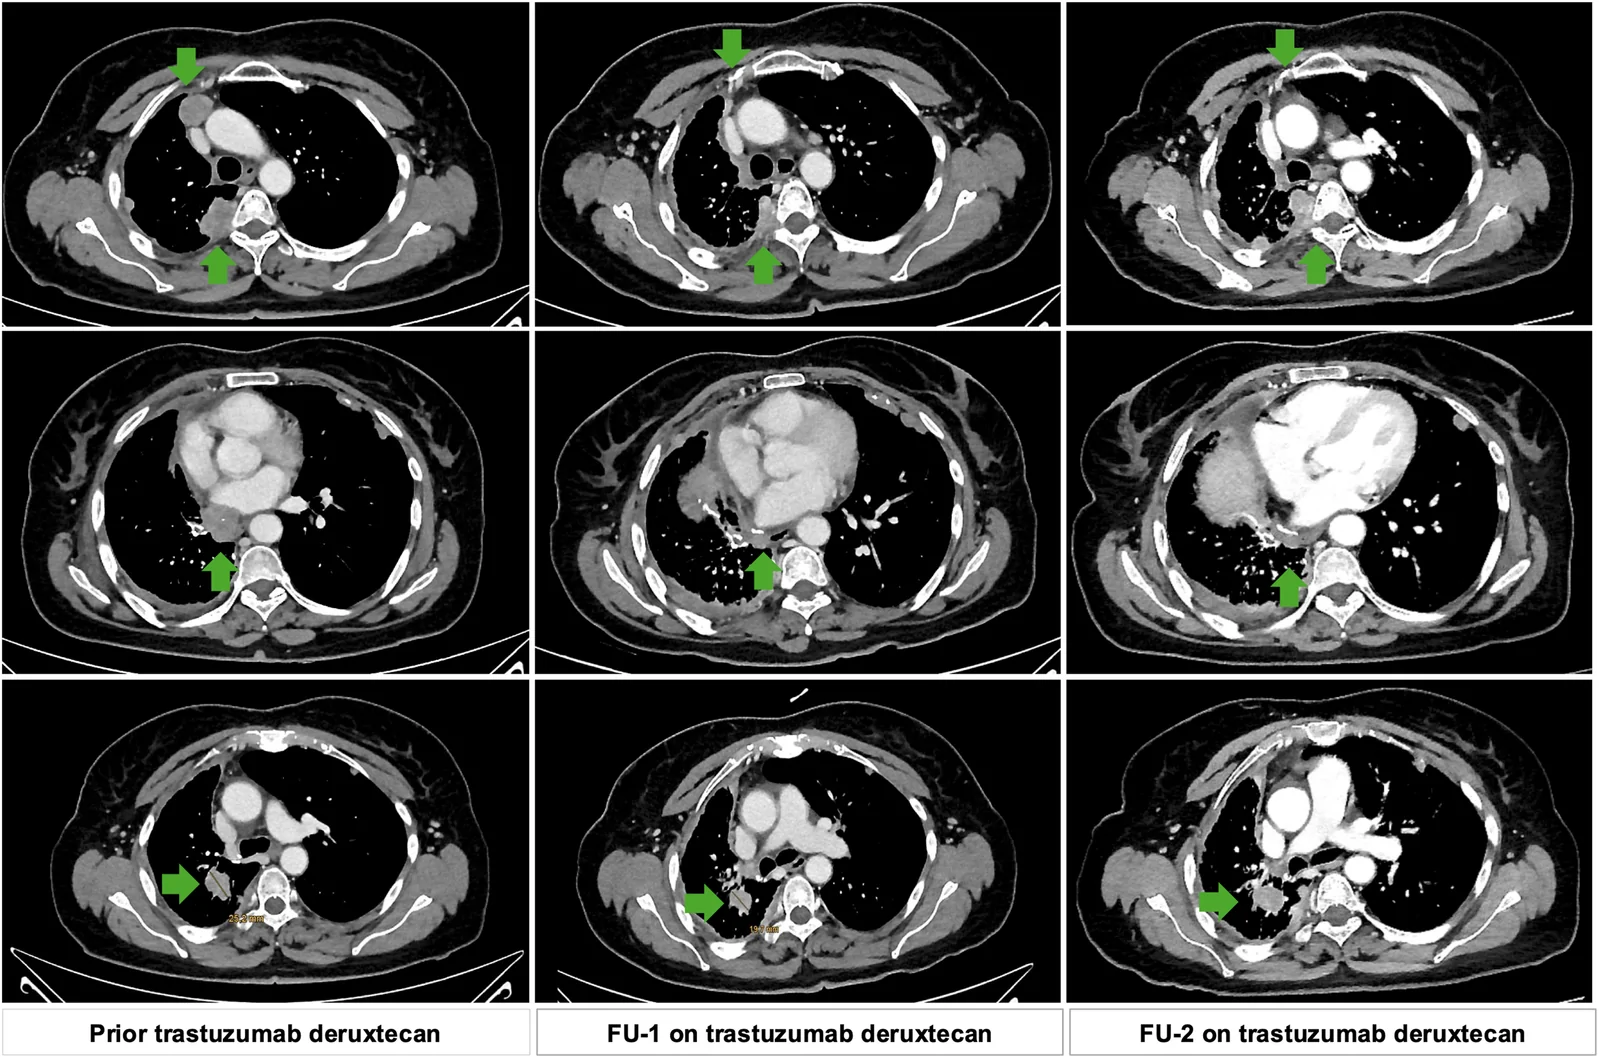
CT scan. Partial response to T-DXd according to RECIST v.1.1. The left column presents the CT scan before T-DXd initiation and the middle and right columns present the staging 8 and 16 weeks after initiation. Tumor shrinkage was observed in all target lesions: mediastinal lymph node (row 1), pleural metastasis (row 1), infrahilar lymph node (row 2), and lung metastasis in the right upper lobe (row 3). CT, computed tomography; FU, follow up; RECIST v.1.1, Response Evaluation Criteria in Solid Tumors version 1; T-DXd, trastuzumab deruxtecan.
